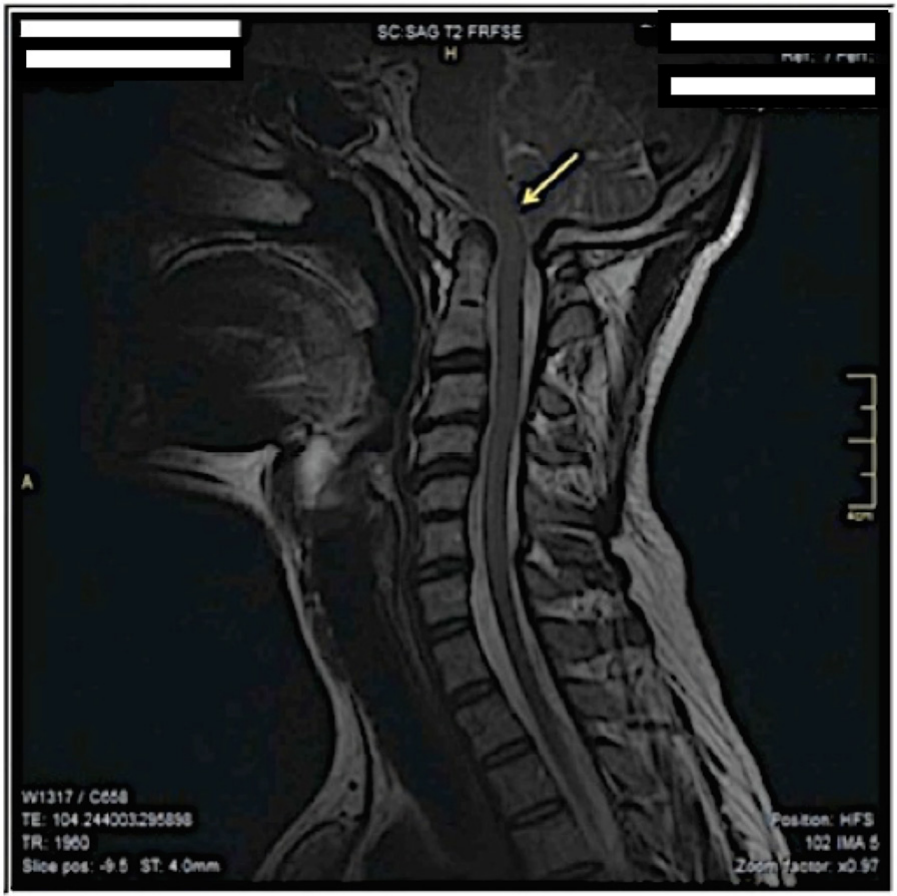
Sagittal T2-weighted MRI. The MRI of the Cranial-cervical structures showing the dorsal increase of the odontoid dens
